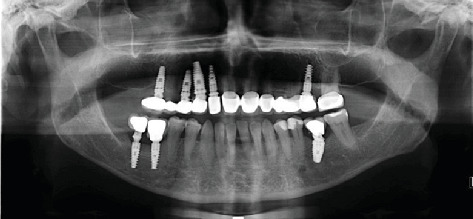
Panoramic radiograph 11 years after implant placement.
